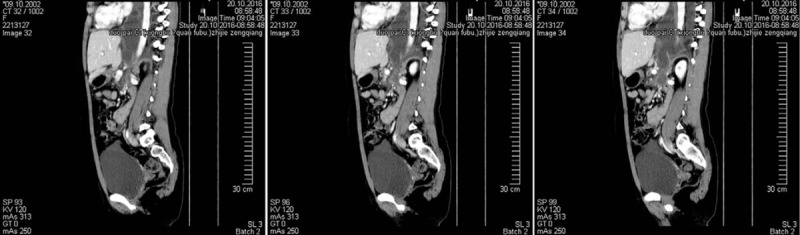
Radiographic examination in the pediatric department of our Hospital. Computed tomography (CT) of the chest and abdomen suggested massive fluid in the bilateral pleural cavities (right greater than left), and a cystic lesion possibility emanating from the tail of the pancreas entering the chest via an esophageal hiatal hernia.
